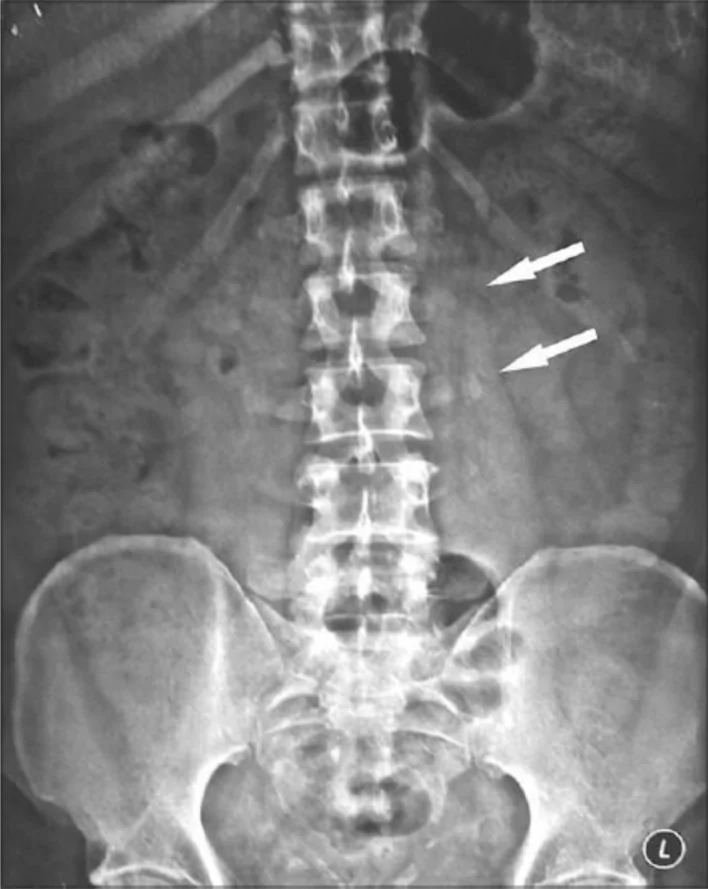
Plain film radiograph of the abdomen and pelvis showing the artefacts masking the fracture line [23]; reproduced from Hegde et al. [23], with permission
